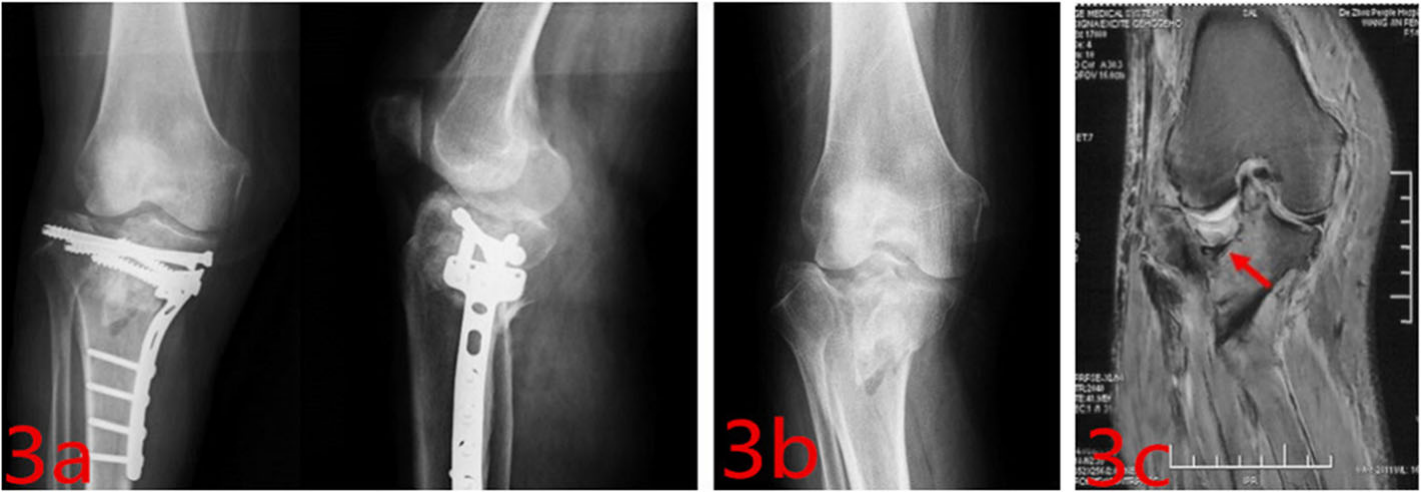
This is a failure case about restoring the width of plateau through the medial approach in other hospital. a Post-operative images showing that the width of plateau was not restored. b Pre-operative images. c The MRI showed the meniscus was trapped in the fracture line
